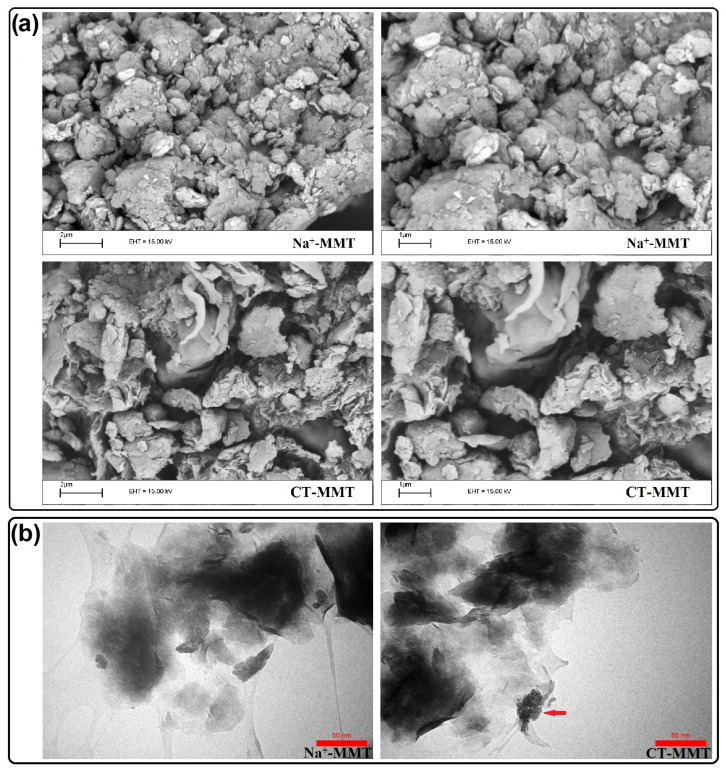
(a) SEM and (b) TEM images of samples Na^+^-MMT and CT-MMT.
